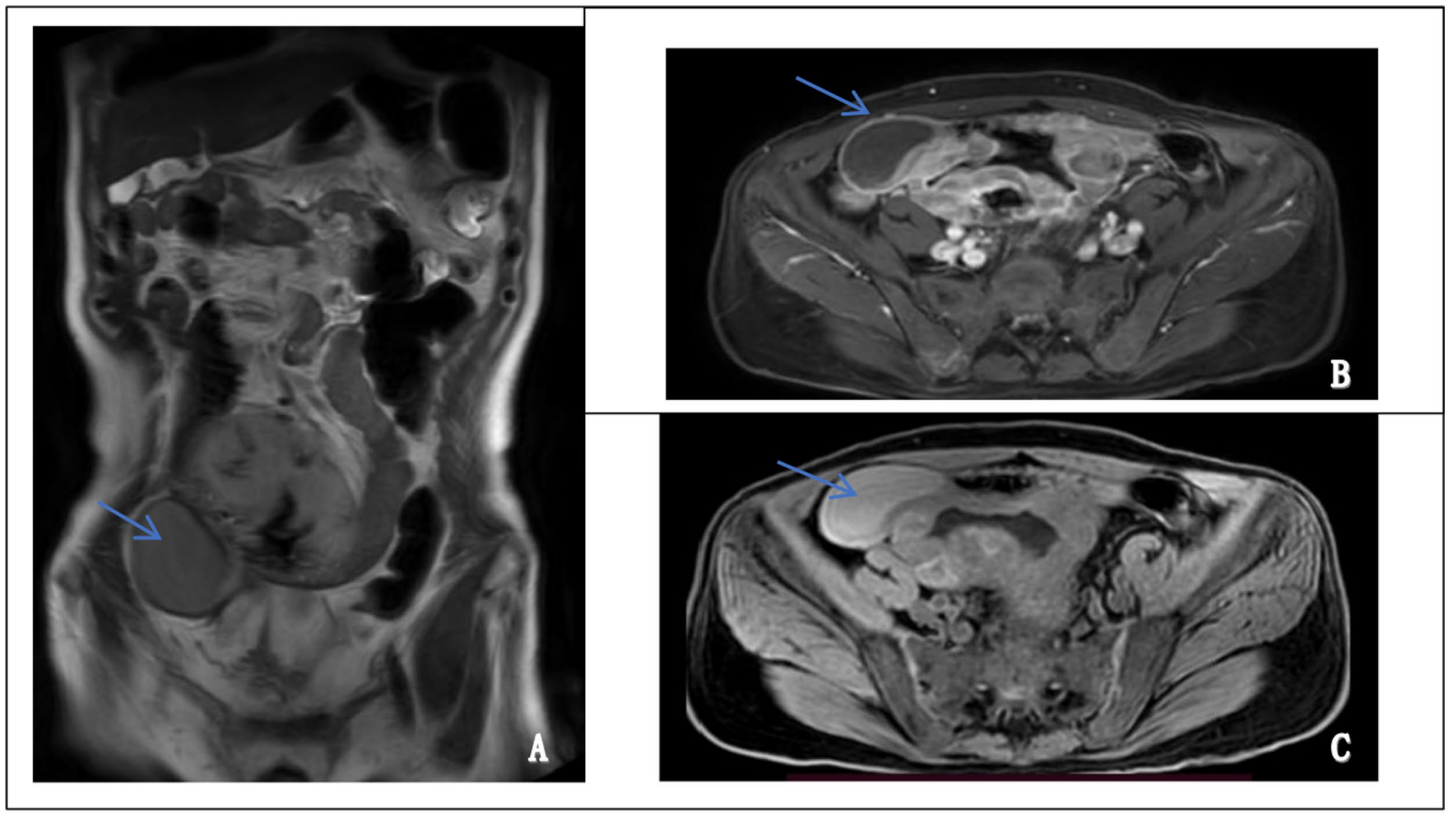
Magnetic resonance imaging (MRI) features of the abdominal mass. (A) Coronal T2-weighted image showing a well-defined, hyperintense mass in the lower abdomen (left panel, arrow). (B) Axial contrast-enhanced image demonstrating heterogeneous enhancement of the lesion, suggestive of a solid, vascularized tumor (upper right panel, arrow). (C) Axial T1-weighted image revealing a hypointense mass at the corresponding site (lower right panel, arrow).
